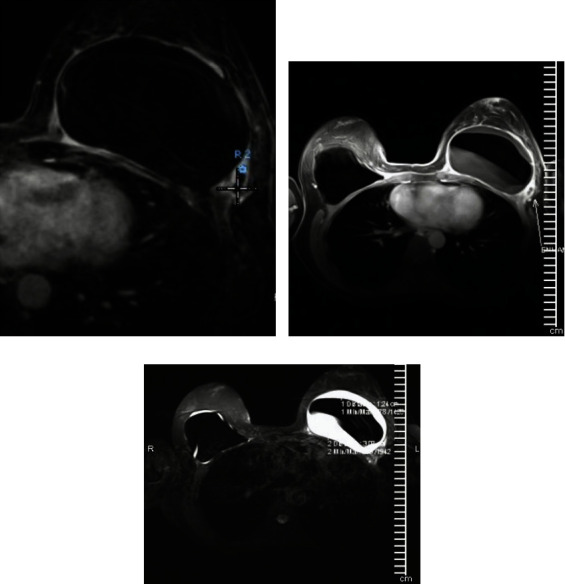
(a–c) MRI images of periprosthetic fluid and mass.
